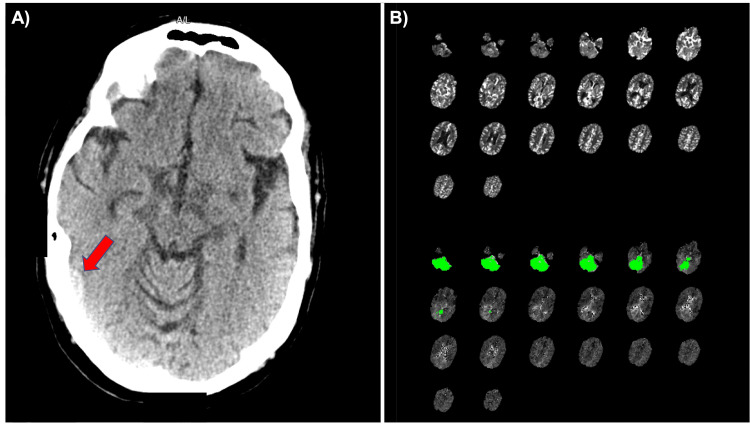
A. CT scan of the brain revealing a small right posterior parietal subdural hematoma. B. CT perfusion scan of the brain revealing ischemia of the cerebellar hemispheres bilaterally with small areas of core infarction and a moderate-to-large surrounding penumbra
